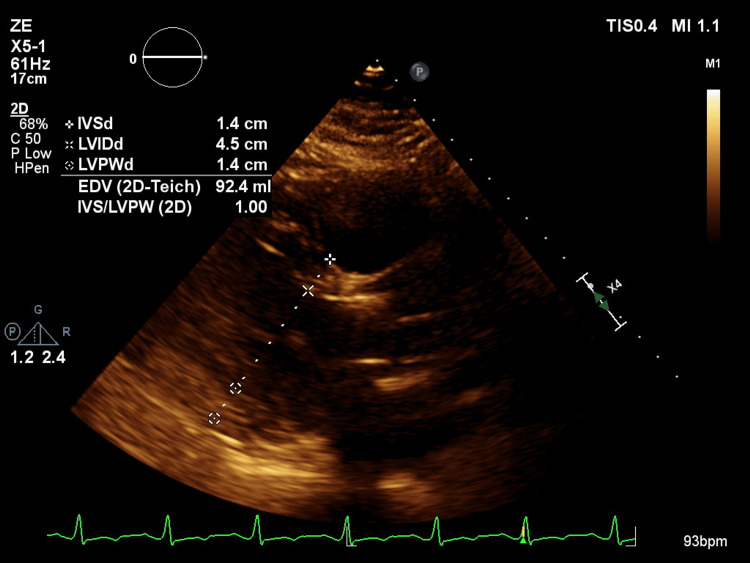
Two-month follow-up post-surgical TTE reflecting improvement of dilated cardiomyopathy and of left ventricular internal diameter during diastole. TTE: transthoracic echocardiography
